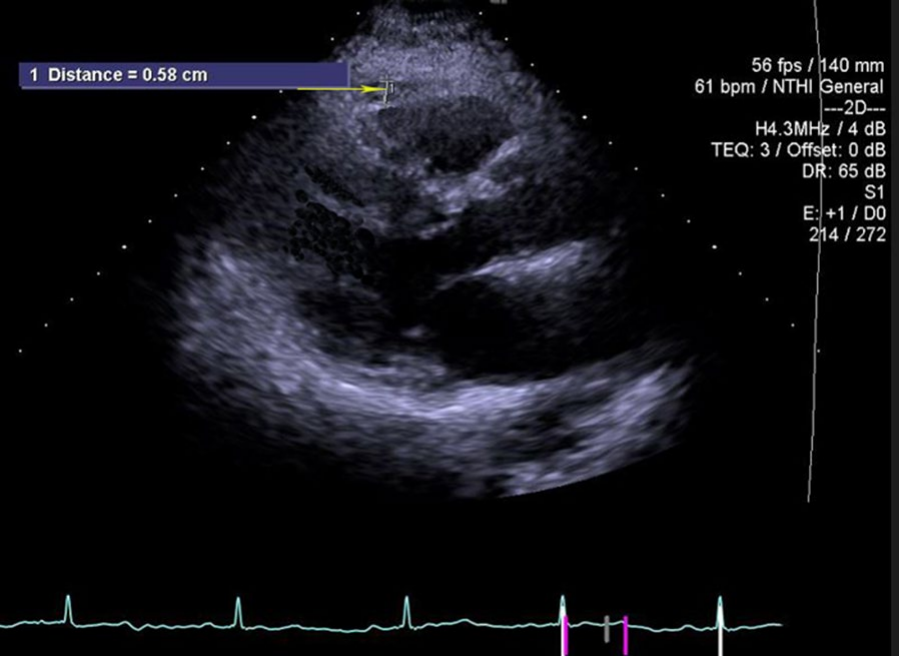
Transthoracic two-dimensional echocardiography in the parasternal long-axis view, showing epicardial fat thickness (arrow)
